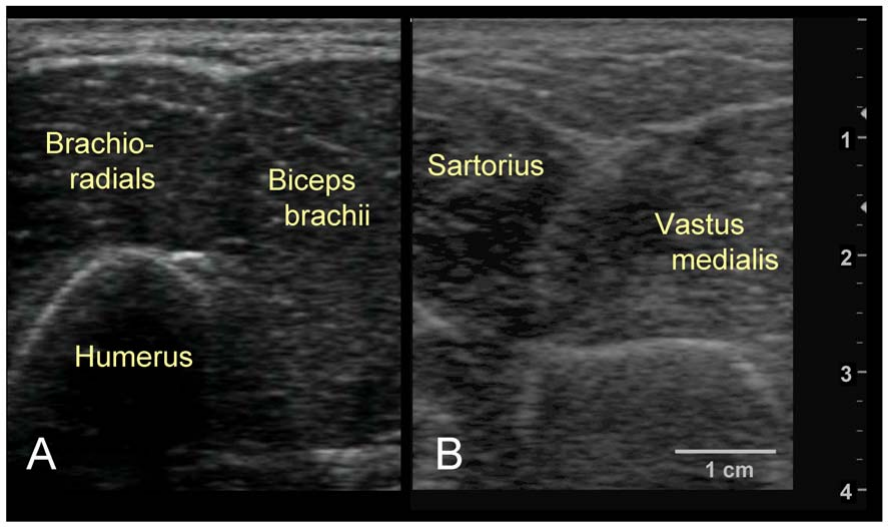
Challenges with Targeting Intermuscular Fascia. (2A.) Ultrasound of upper arm. There are frequently no clear distinctive divisions between brachioradialis and biceps brachii muscles as shown in this ultrasound image. (2B.) Ultrasound of leg. The fascia between the sartorius and vastus medialis muscles have oblique depth angles.
